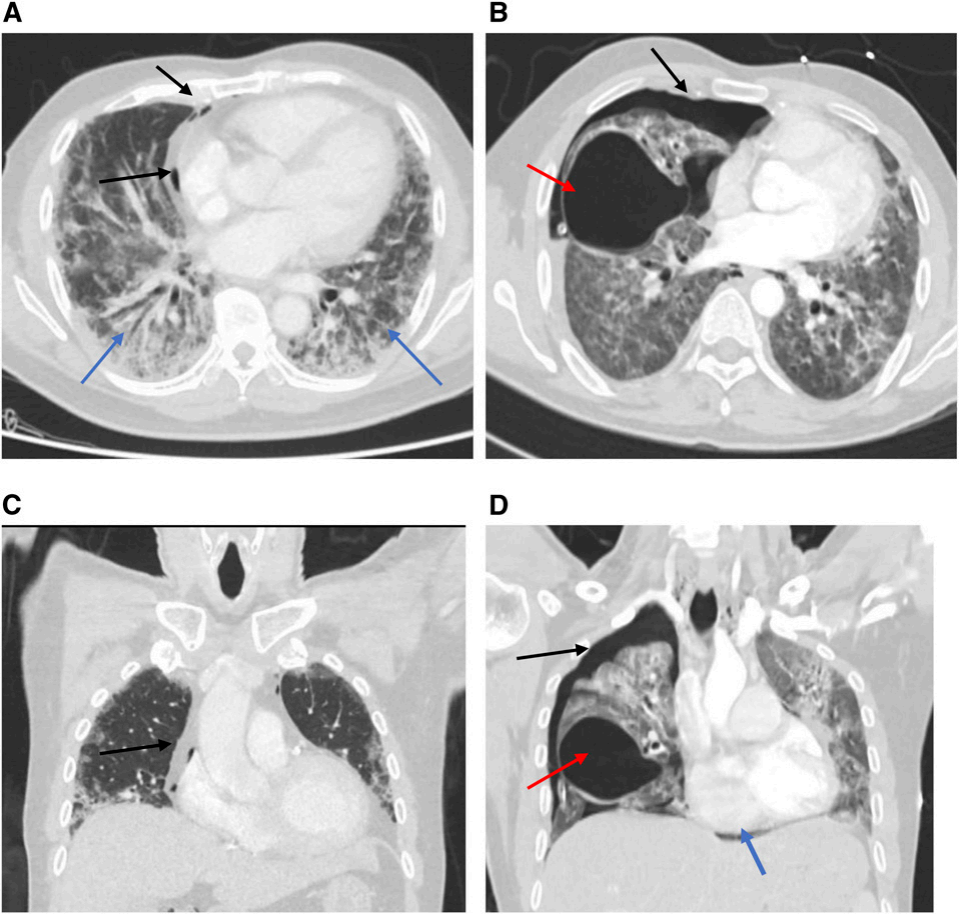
Computed tomography scans of patients diagnosed with COVID-19. (A and B) Axial and coronal plane of a chest CT scan of case 1 showing bilateral infiltrates (blue arrows) and pneumomediastinum (black arrows). (C and D) Axial and coronal plane of CT thorax of case 2 showing a moderate right-sided pneumothorax (black arrow) and large air containing bulla right middle lobe (red arrow). The mediastinal structures are shifted to the left with mild pneumomediastinum noted (blue arrow).
